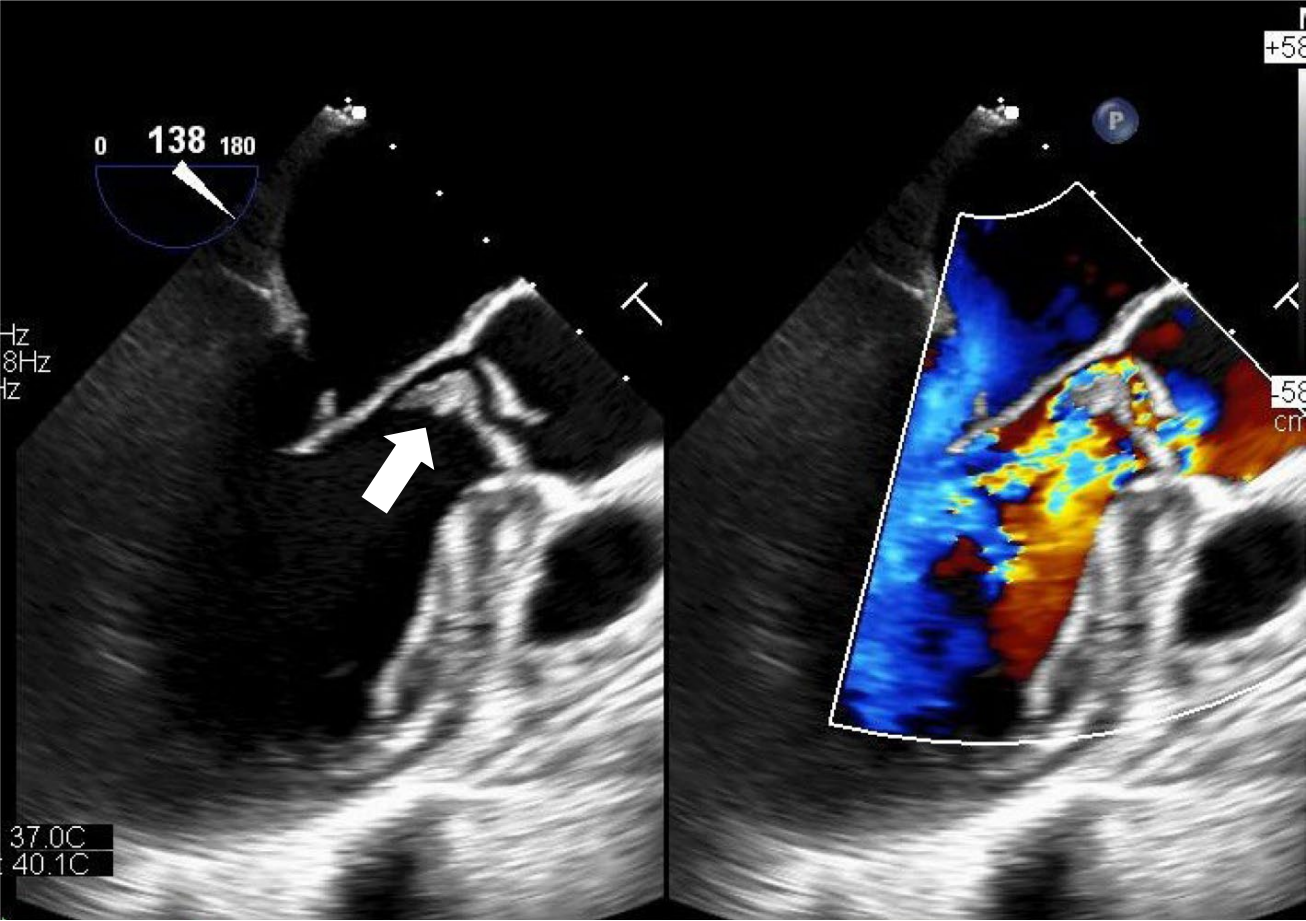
Transesophageal echocardiogram findings of the aortic valve. A large vegetation (20 × 12 mm) on the right coronary cusp leaflet, which reciprocates between the aorta and left ventricle with cardiac contraction, is observed (arrow) (left). Severe aortic regurgitation is noted (right)
